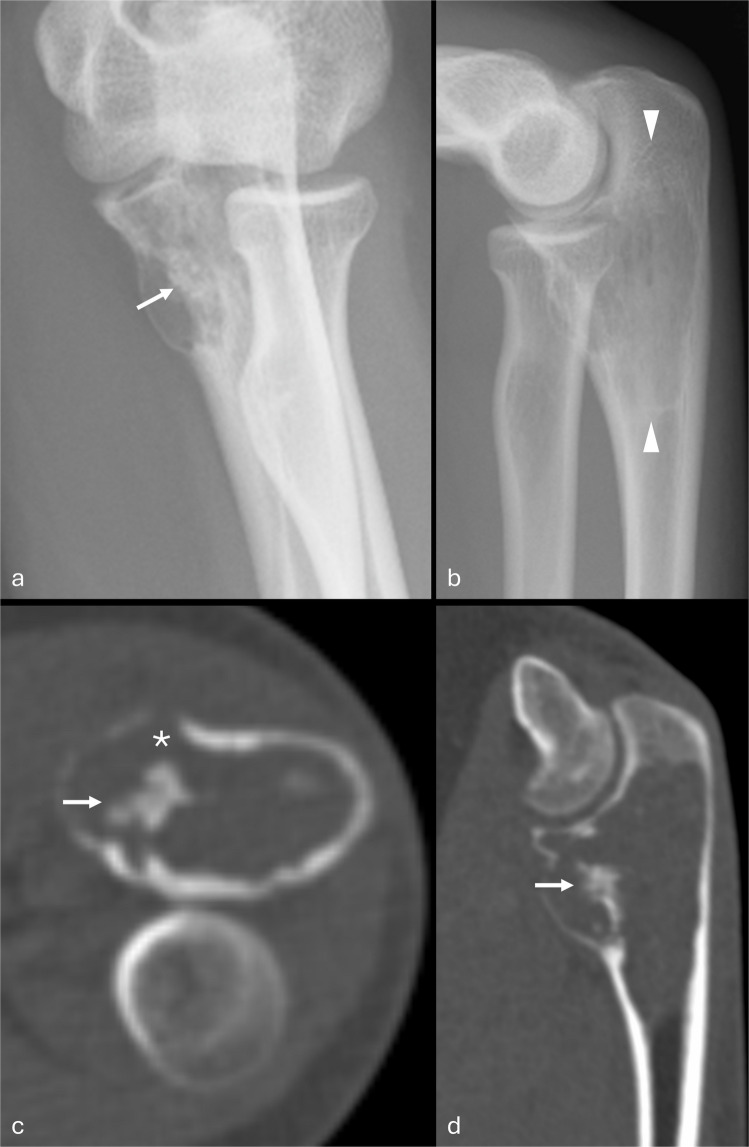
Case 2. AP (a) and lateral (b) radiographs of the left elbow. A lucent lesion is seen in the proximal ulna. There is mild expansile remodeling, and the anterior cortex is markedly thinned. A focus of increased density is identified (arrow, a), not seen on the lateral radiograph. The lesion is poorly defined (arrowheads, b) and slightly lobular. Axial (c) and sagittal (d) CT reformats show a focus of ossification in the anterior aspect of the lesion (arrows), marked thinning of the anterior cortex, and a small cortical breach (asterisk, c). There is lobular morphology with endosteal scalloping (d)
